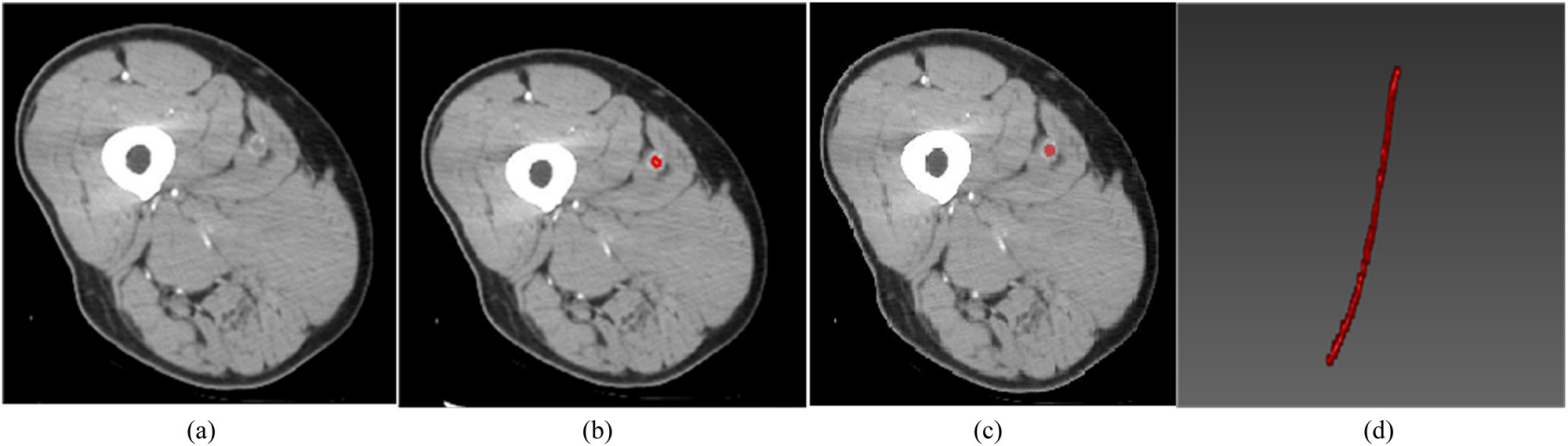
Example of 3D ROI segmentation. Male patient aged 65 years with thrombosis in the right lower extremity deep vein. (a) CTA imaging. (b and c) Image segmentation performed on CTA images. (d) 3D ROI segmentation.
